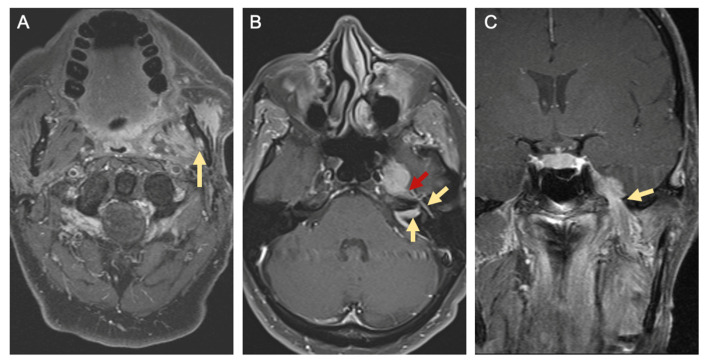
Radiographic signs of perineural spread. T1-weighted magnetic resonance (MR) images with gadolinium from a patient with left buccal squamous cell carcinoma and perineural invasion. (A) Axial image showing enlargement and enhancement of the left inferior alveolar canal (yellow arrow). (B) Axial image showing enhancement of the intracanalicular and tympanic segments of the facial nerve (yellow arrows) as well as the greater superficial petrosal nerve (red arrow). (C) Coronal image showing enlargement and tumor involvement of the mandibular branch of the left trigeminal nerve at the foramen ovale (yellow arrow).
